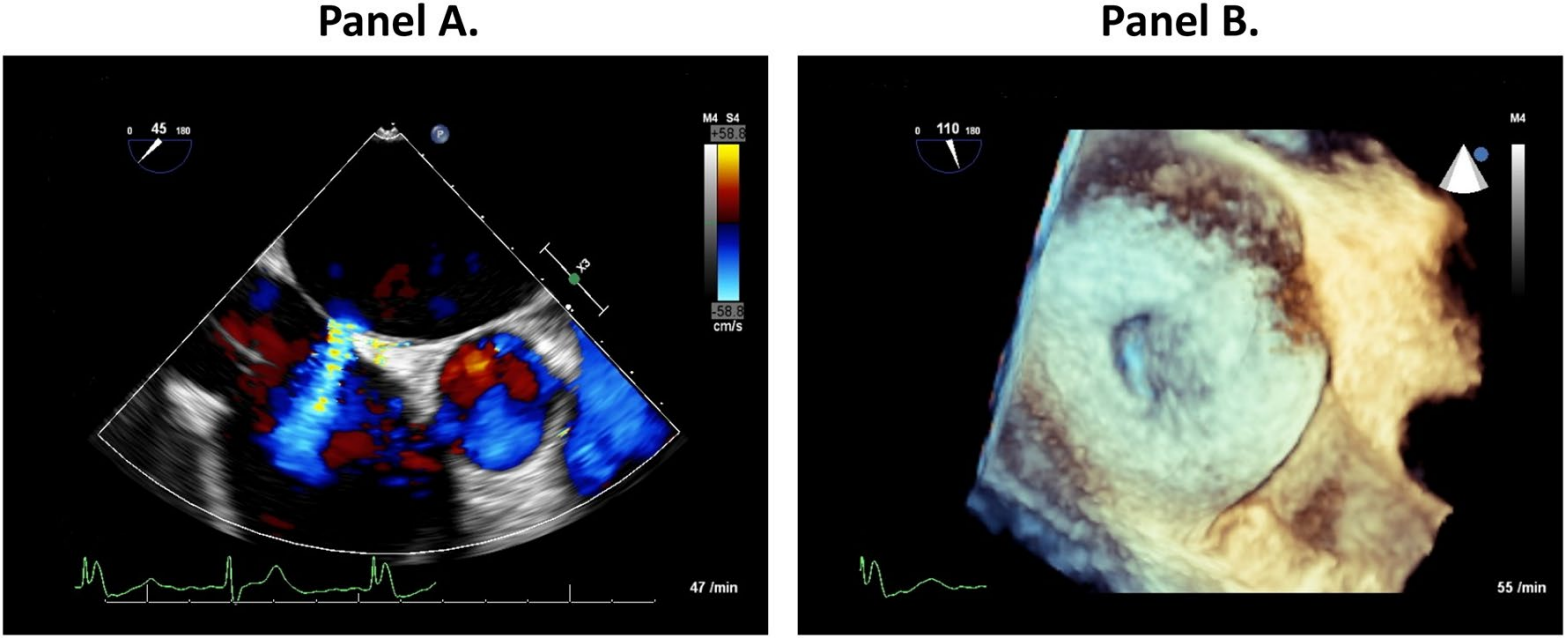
Iatrogenic atrial septal defect (iASD) at 6 months follow-up after MitraClip procedure. Panel (A) Transesophageal echocardiography with colour Doppler jet showing left to right shunting. Panel (B) 3D transesophageal echocardiography direct en-face imaging of the area of the iatrogenic atrial septal defect.
